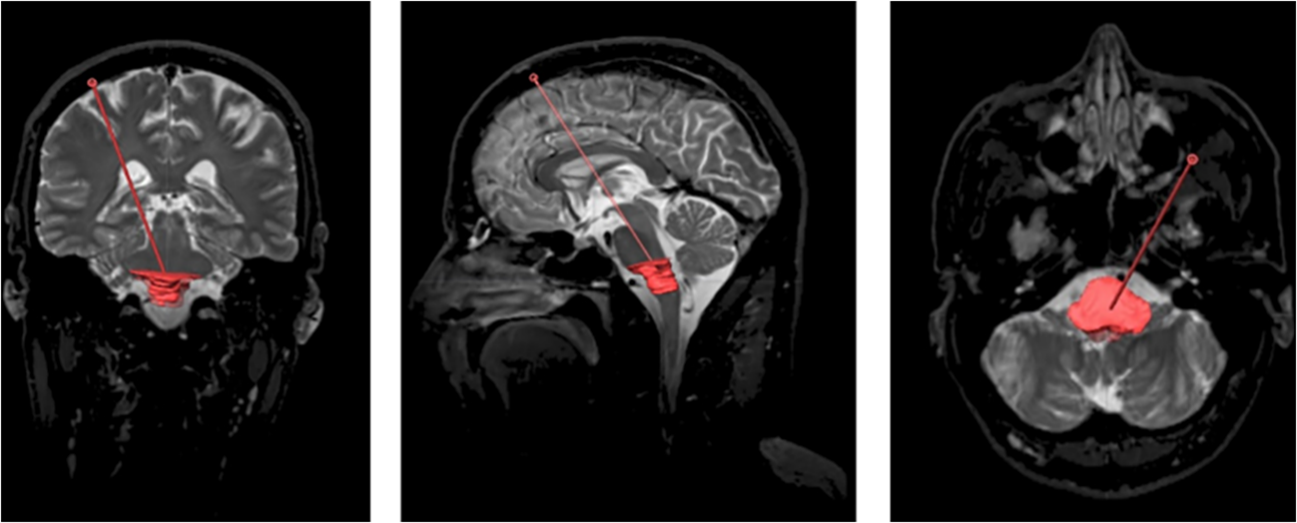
Coronal (left), sagittal (middle), and axial (right) T2-weighted MRI showing the medulla oblongata region of interest (ROI) and the proposed trajectory
